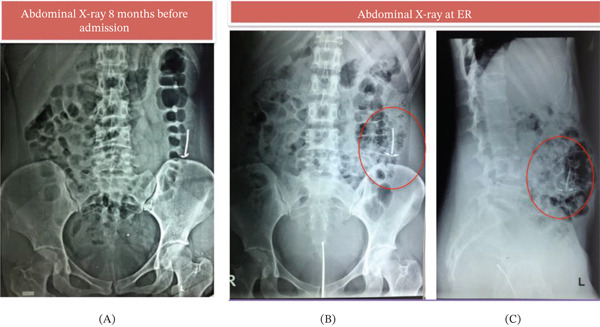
Plain abdominal x‐ray demonstrating the extrapelvic migration of the intrauterine device (IUD). (A) AP projection of abdominal radiography 8 months before the admission. (B) AP and (C) left lateral projection with the uterine marker at emergency room.
